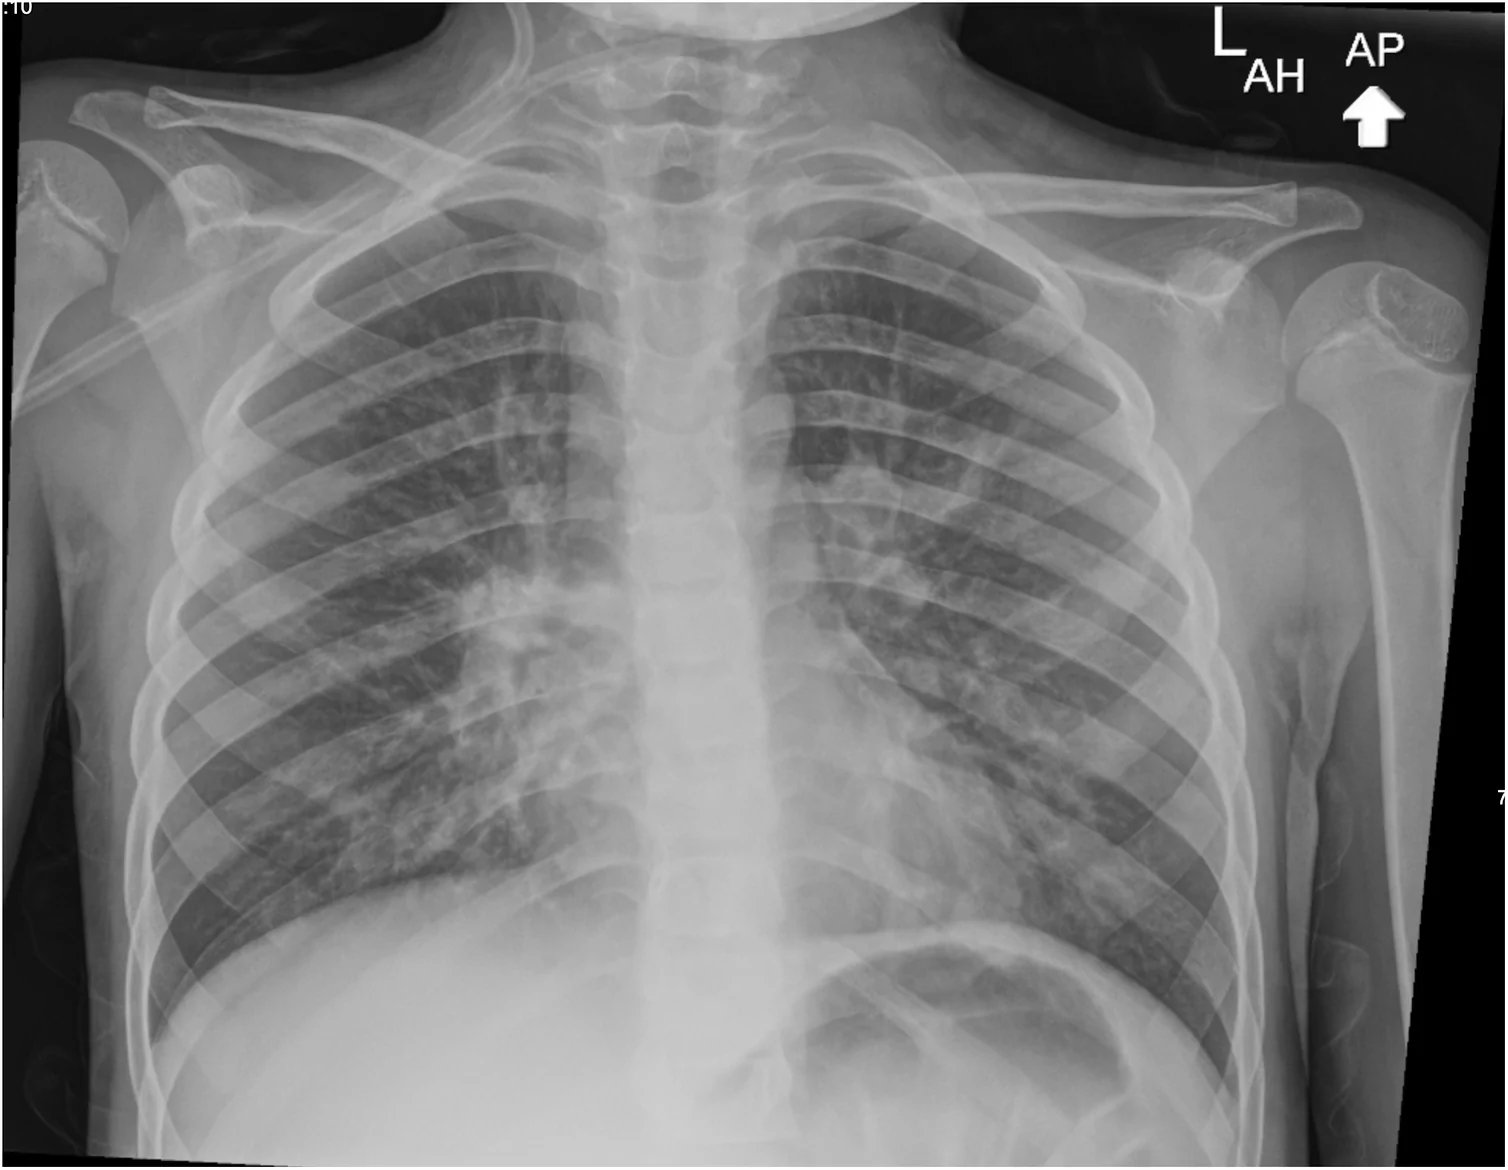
Chest radiograph - anteroposterior chest radiograph demonstrating right middle lobe opacity consistent with atelectasis and bilateral peribronchial thickening.
